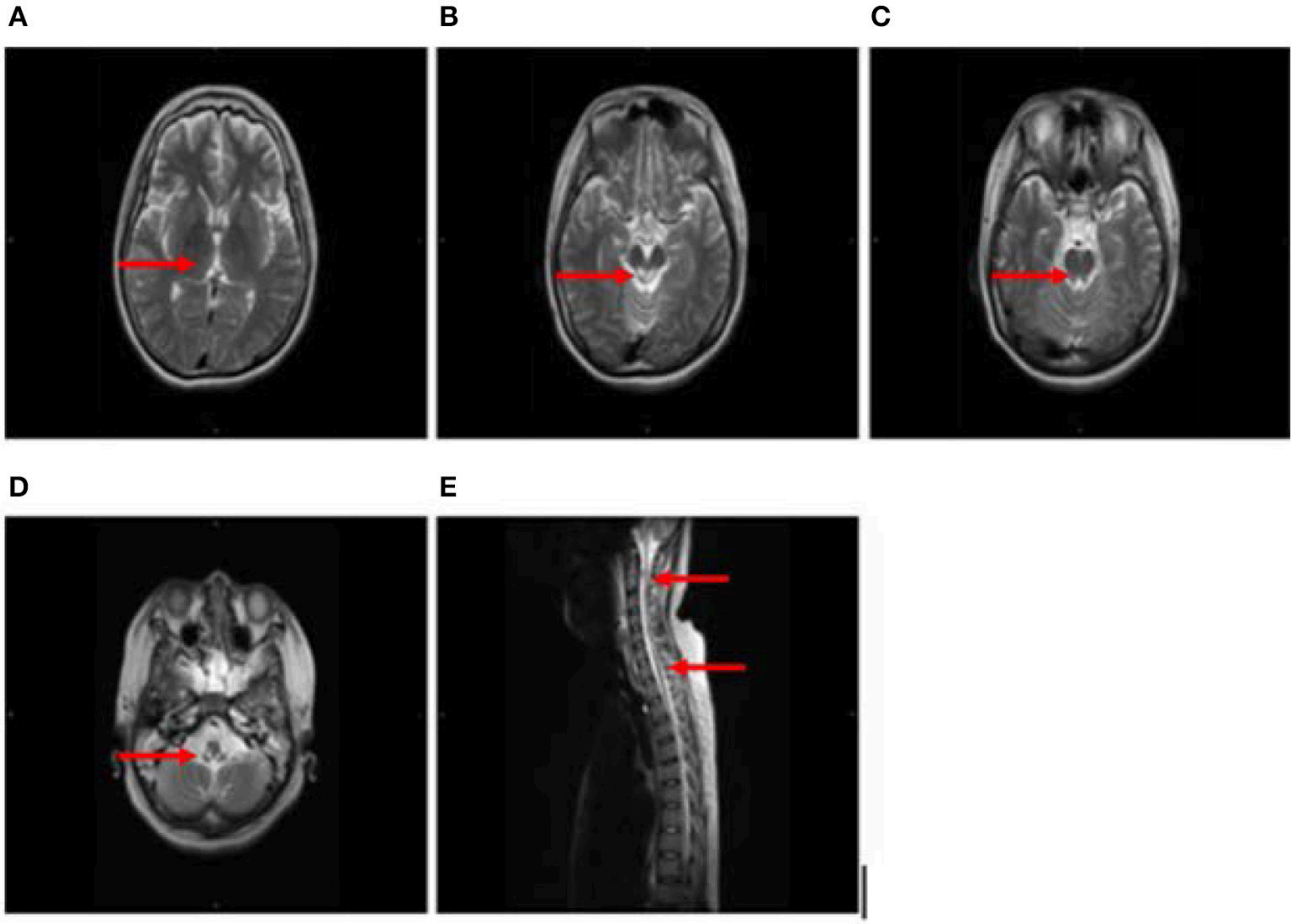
(A–E) T2-weighted MRI results at 8 months following hospitalization were similar to those obtained 1 month earlier (arrow in A–E).
